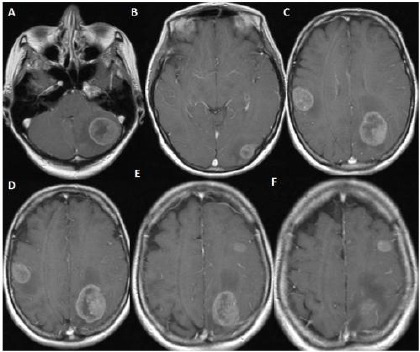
A, B, C, D, E, F (Table 1: Case 5, Metastatic lung tumour): Gadolinium-enhanced T1 weighted axial magnetic resonance image scans showing seven different metastatic lesion with a heterogeneous intensity. I extracted three of seven lesions, because three of them larger than 3 cm on each scan and smaller lesions were left for gamma knife surgery. The first one of the extracted tumour located at the left side of the posterior fossa and it was extracted through conventional craniotomy technique, so it is out of interest of this article. The second one of them located at the right motor cortex. And the third one of them located at the medial part of the left parietal lobe. The two ones were extracted using stereotaxy*.
